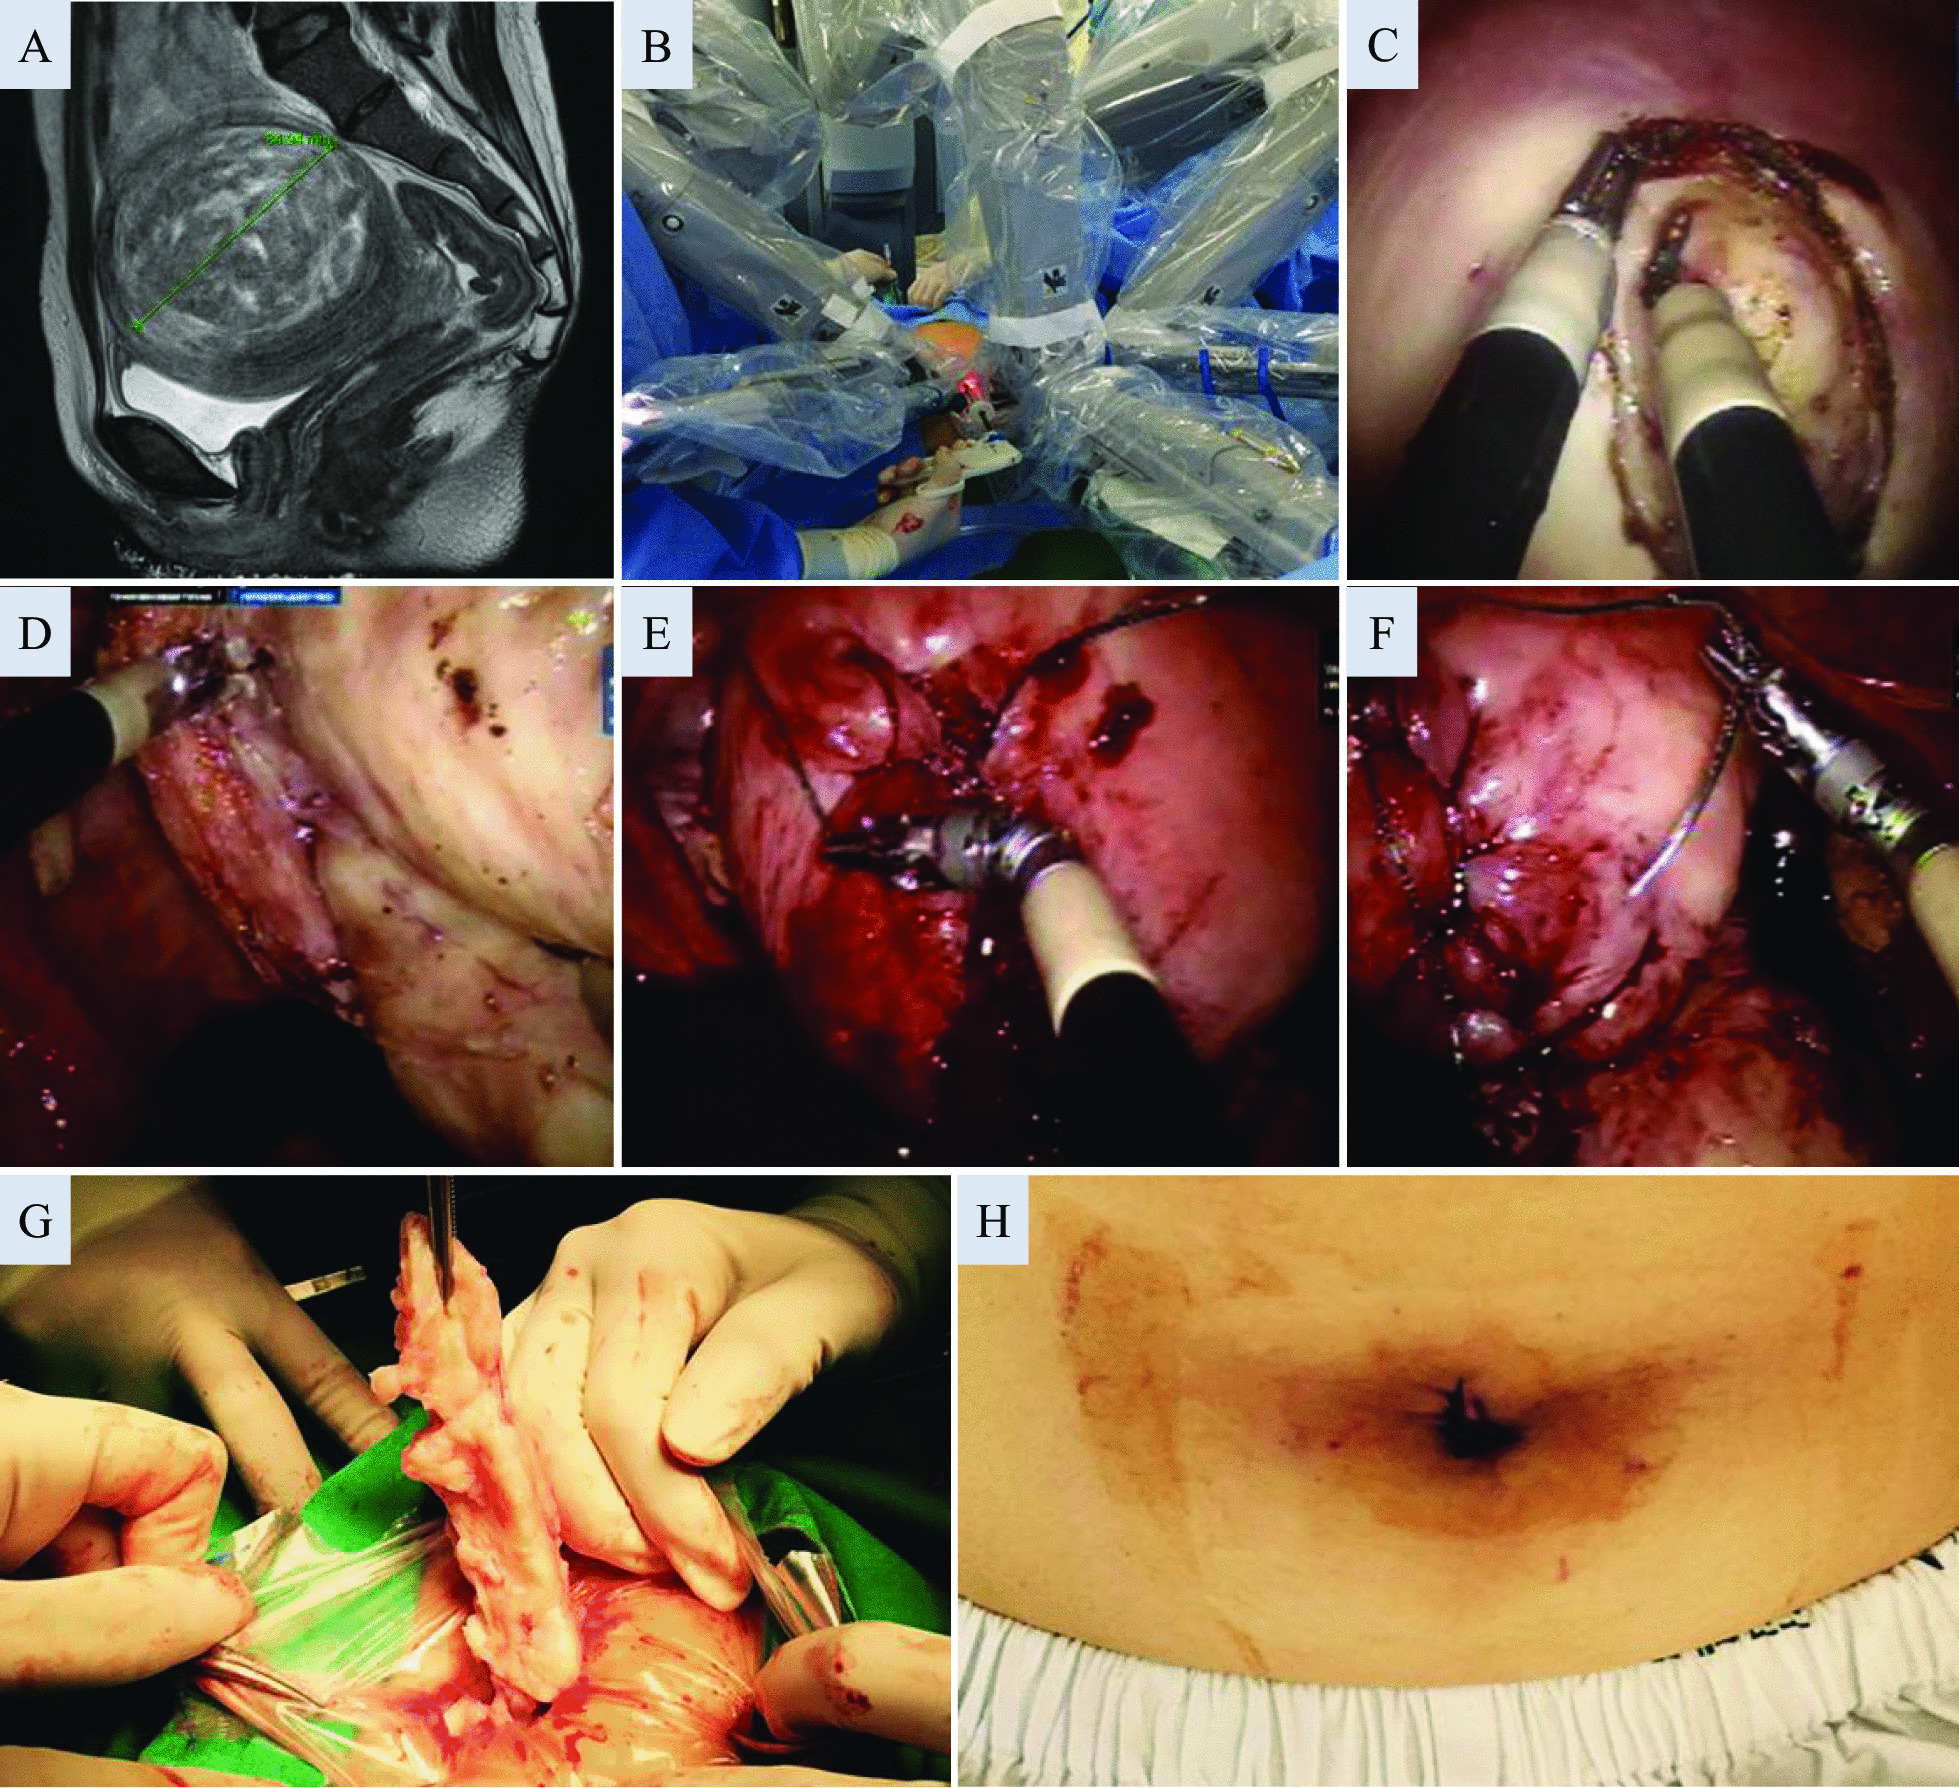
A stepwise description of a representative robotic single-site myomectomy case. Myomectomy was recommended for a 32 year-old woman due to menorrhagia and infertility for a period of 14 months. To decrease the size of this 8.5-cm FIGO type 3 myoma, leuprolide acetate was injected, but surgery could not be postponed due to intolerable heavy vaginal bleeding for 3 weeks. A Due to the unusual amount of bleeding and suspected distortion of the uterine cavity on ultrasonography, a pelvic magnetic resonance imaging was performed prior to the surgery (T2-weighted image). B A view of the operating field after docking of the Da Vinci Si single-site platform, with the first assistant to the right of the patient controlling the assistant port. The maintenance of this configuration of the robotic arms is important to create adequate triangulation intra-abdominally and to minimize tool clashing. C The myometrial incision was placed with a monopolar hook. D Although the robotic tools for the single-site platform have less variety, skillful use of bipolar forceps, the hook, and/or the wristed needle driver allows for adequate dissection. E Suturing was performed with V-locks in three layers, involving deep myometrial sutures to achieve adequate approximation and to prevent dead spaces from forming within the myometrium. F Although the serosal layer is most commonly approximated with Vicryls, when barbed suture materials are used, continuous sutures are placed so that the barbing is not exposed, and an anti-adhesive film is covered at the end. G Manual in-bag morcellation of the myoma with spiral incisions through the 2.5-cm umbilical port. H The 2.5-cm umbilical incision immediately after closure
